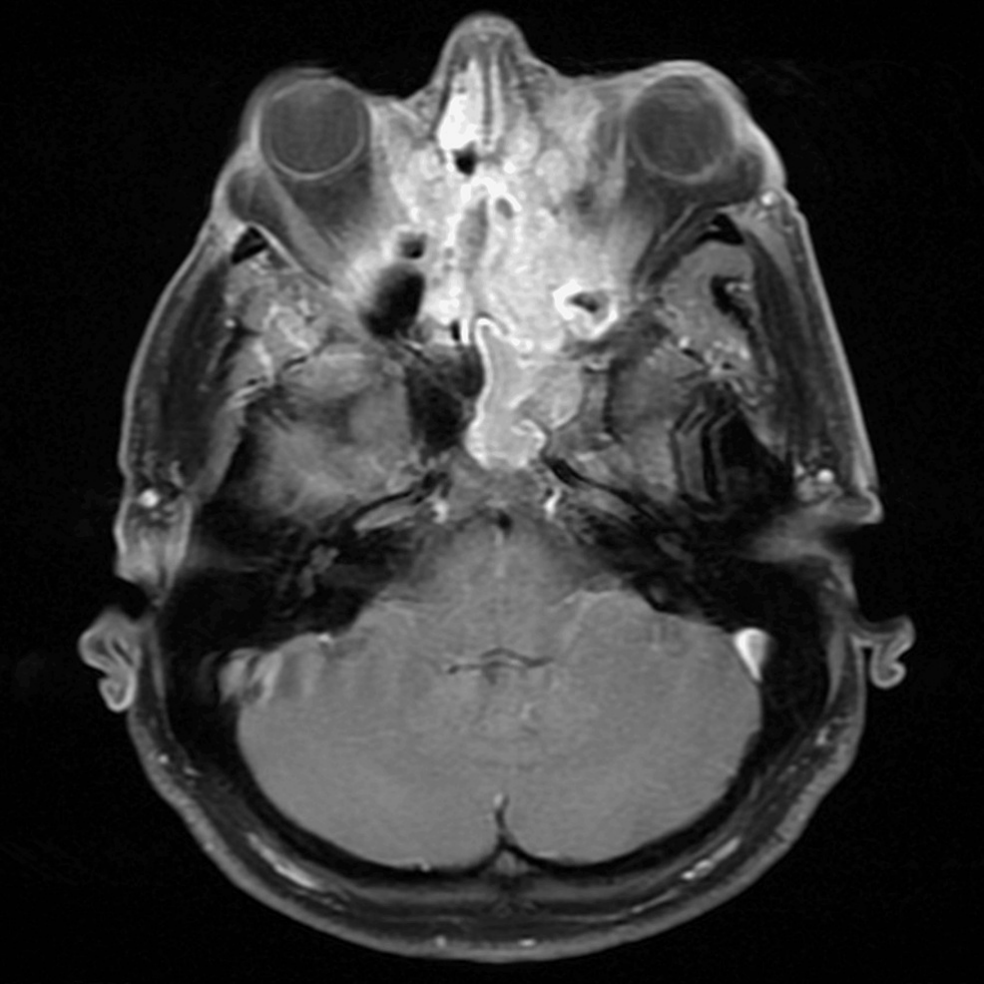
CT image of an esthesioneuroblastoma, depicted as a large enhancing mass centered on the midline of the anterior cranial fossa and involving both orbits. Image Credit: Frank Gaillard, 2010 [14]; Creative Commons License
